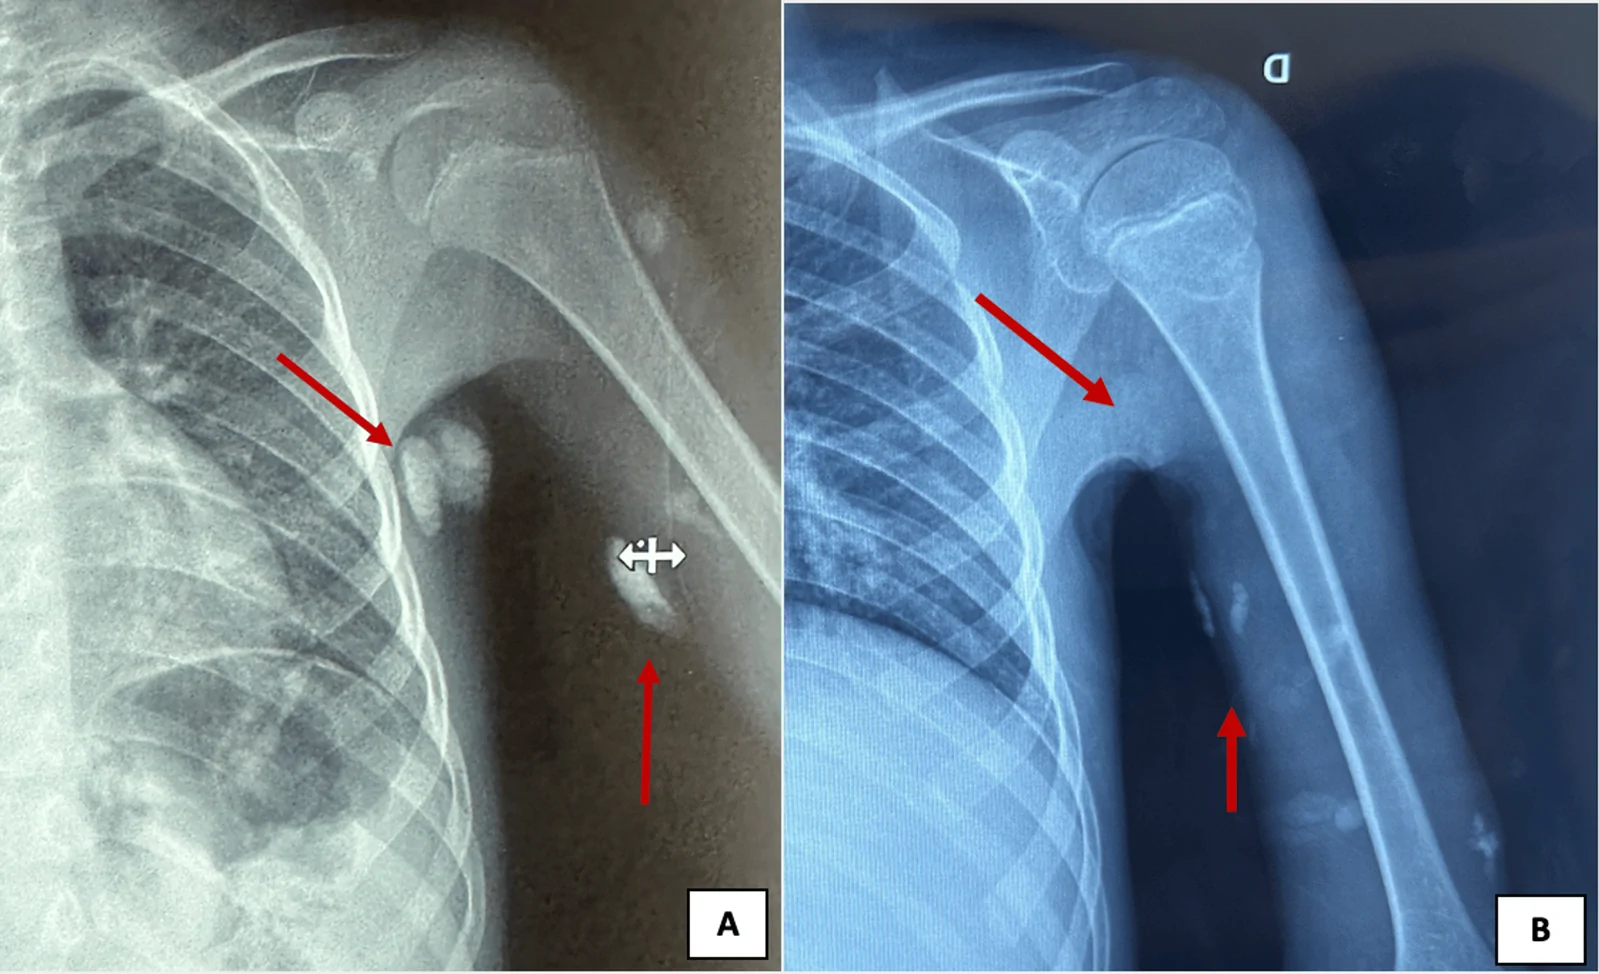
Radiological improvement after treatment. (A) Plain radiograph of the upper limb before treatment showing extensive radiopaque calcified deposits within the soft tissues of the arm (red arrows). (B) Follow-up radiograph after six months of treatment demonstrating marked regression of the calcified deposits (red arrows).
